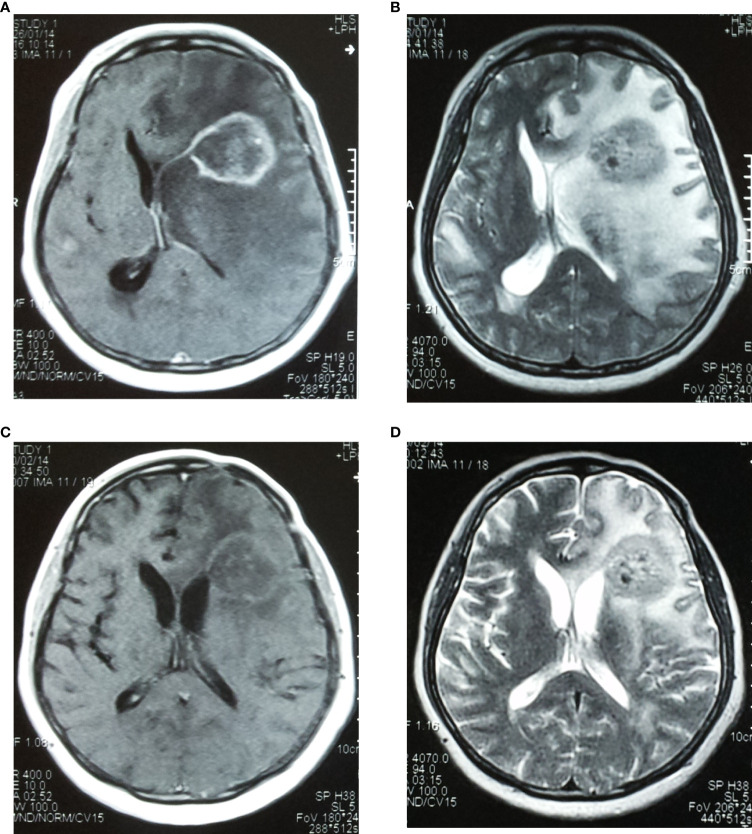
A case of brain metastasis derived from colon cancer with radiation-induced brain necrosis in the left frontal lobe that was then treated with 5 mg/kg bevacizumab. (A) Before treatment, MRI T1 showed enhanced areas of brain necrosis; (B) Before treatment, MRI T2 showed obvious perifocal oedema. (C, D) Two weeks after treatment, the area of oedema was obviously decreased, and the quality of life of the patient was significantly improved.
